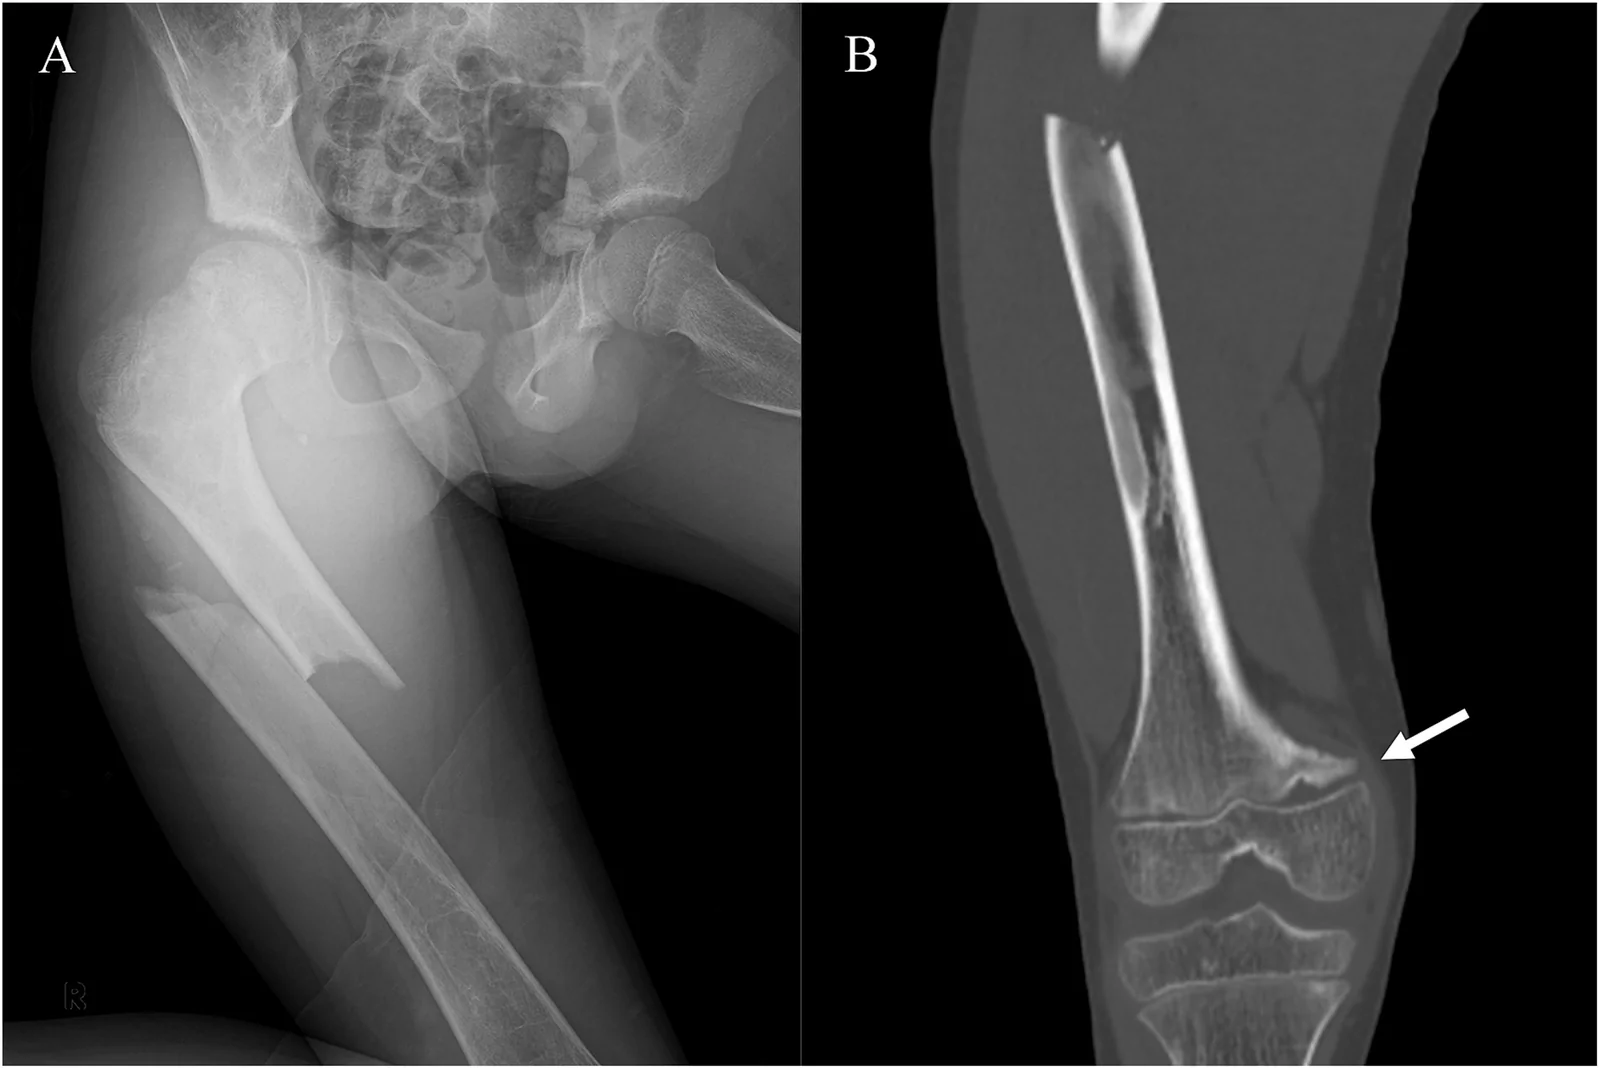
Pathological fracture and persistent rachitic changes in Case 2 at age 11. (A) Anteroposterior radiograph showing a right femoral pathological fracture. (B) Coronal CT image showing distal femoral physeal widening and irregularity with adjacent metaphyseal fraying, compatible with persistent rachitic change. The arrow indicates the distal femoral metaphyseal/physeal abnormality.
